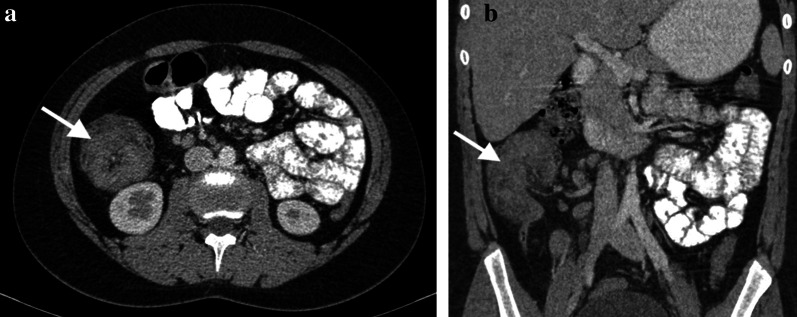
a, b A 13-year-old boy with Burkitt lymphoma. The patient had abdominal pain and vomiting for the last 15 days. Invagination was detected in US performed in an external center. Axial and coronal reformatted CT images show intussusception at the level of the ileocecal valve (arrows)
